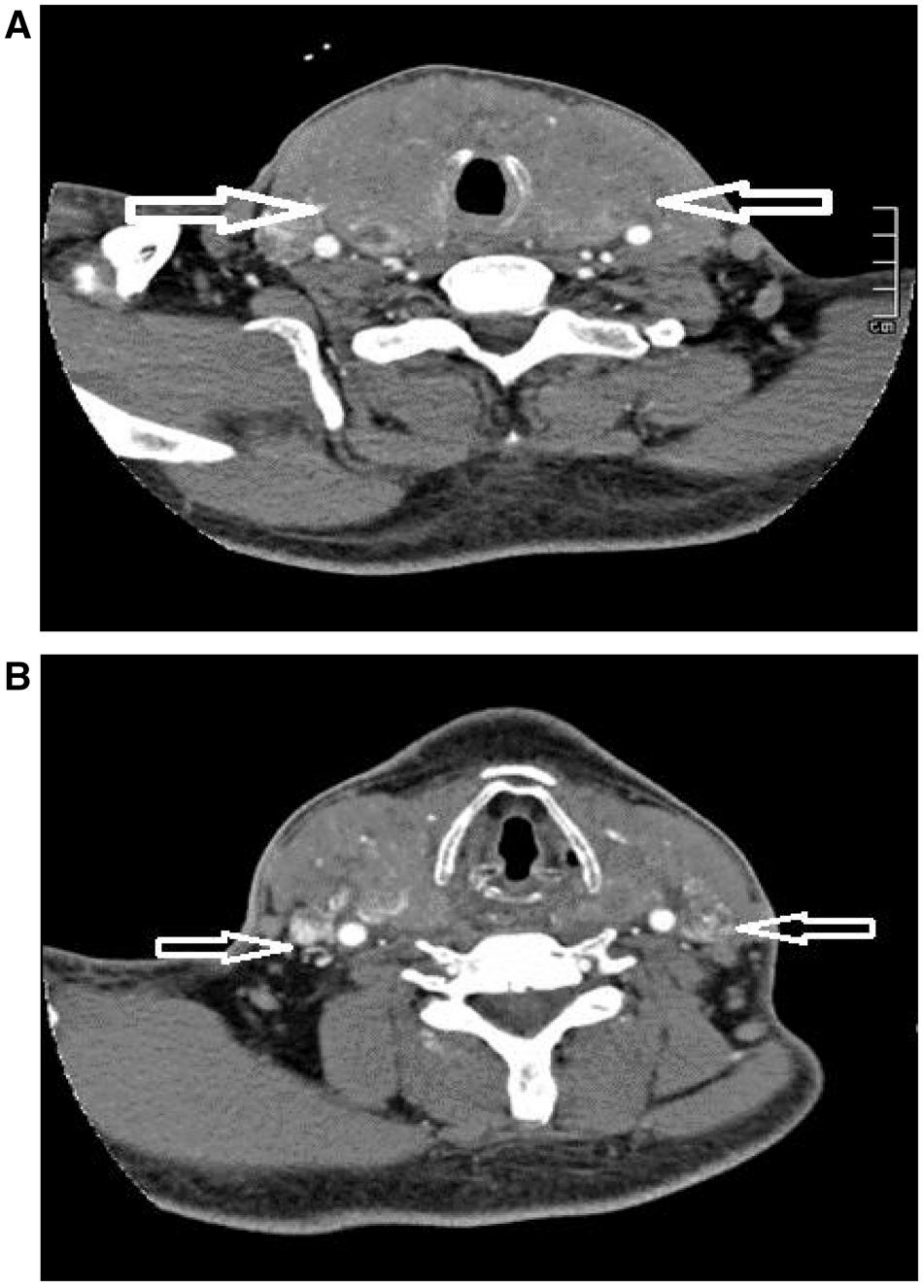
(A) Enhanced CT examination showed markedly enlarged thyroid volume and uneven enhancement of bilateral lobes, with circular and nodular calcified foci visible within. (B) Slightly hypodense shadows and nodular and irregularly shaped hypodense shadows are seen filling the lumen of the bilateral jugular vein tracts.
